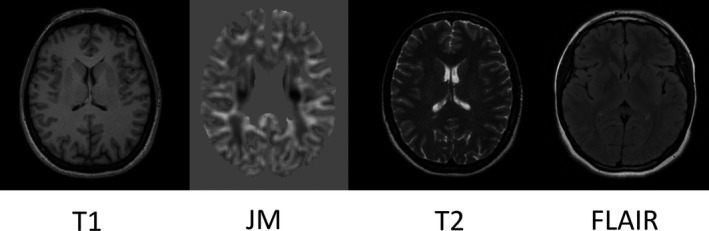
Examples of the magnetic resonance (MR) or MR‐derived image volumes used in the study: a T1‐weighted image volume, a junction map (JM),^13^ a T2‐weighted image volume, and a fluid‐attenuated inversion recovery (FLAIR) volume
